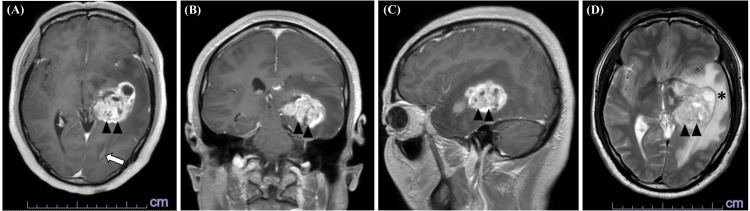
Preoperative MRI findings Initial brain MRI demonstrated a ring-shaped enhanced mass lesion involving the left medial temporal lobe (arrowheads) extending to the wall of the lateral ventricle (arrow) on post-contrast T1WIs of axial (A), coronal (B), and sagittal (C) sequences. The tumor exhibited perifocal edema on T2WI (D, asterisk). MRI: magnetic resonance imaging; WI: weighted image
